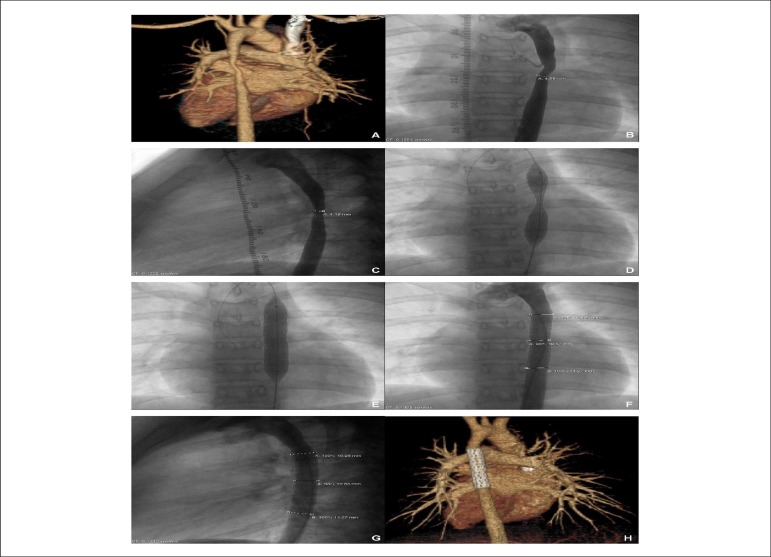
Computed tomography (1A), frontal thoracic aortography (1B), and lateral
